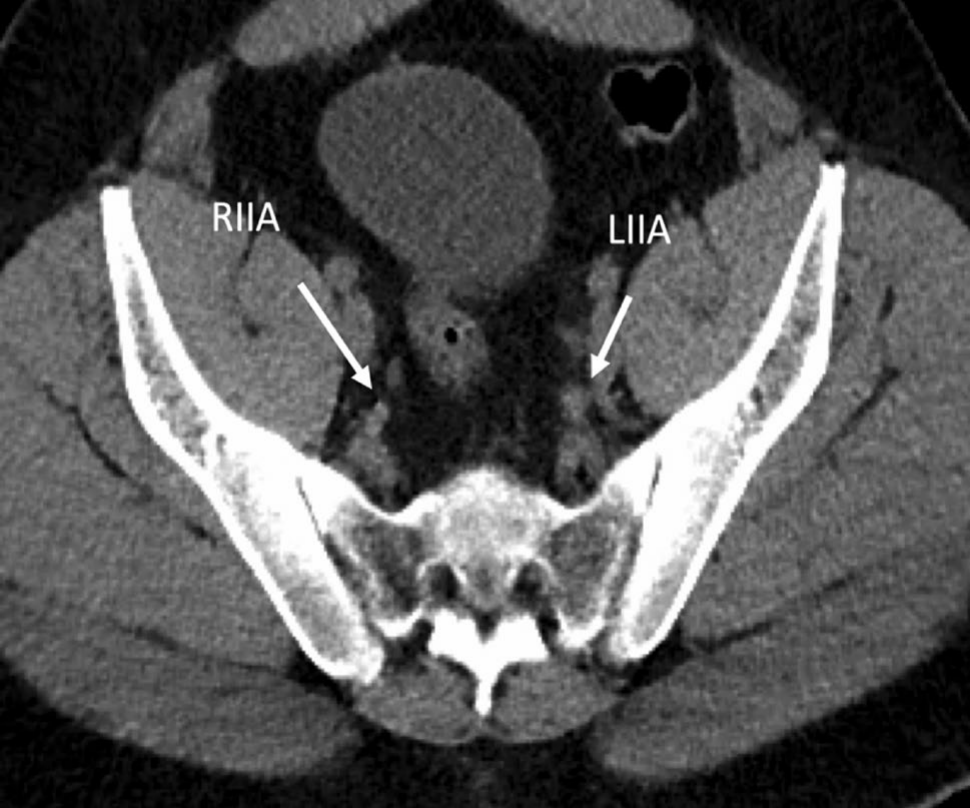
Non-contrast CT images of a 41-year-old male non-smoker, who scored 0/10 on calcium scoring. No arterial vascular calcification was demonstrated. RIIA/LIIA right internal iliac artery/left internal iliac artery
